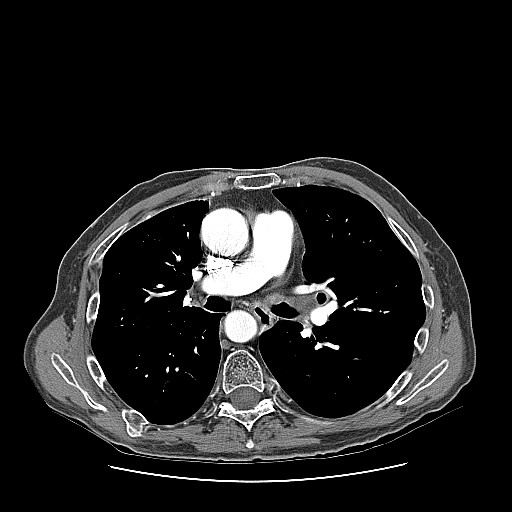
Computer tomography (CT) of the chest showing soft tissue thickening around LMS bronchus.
